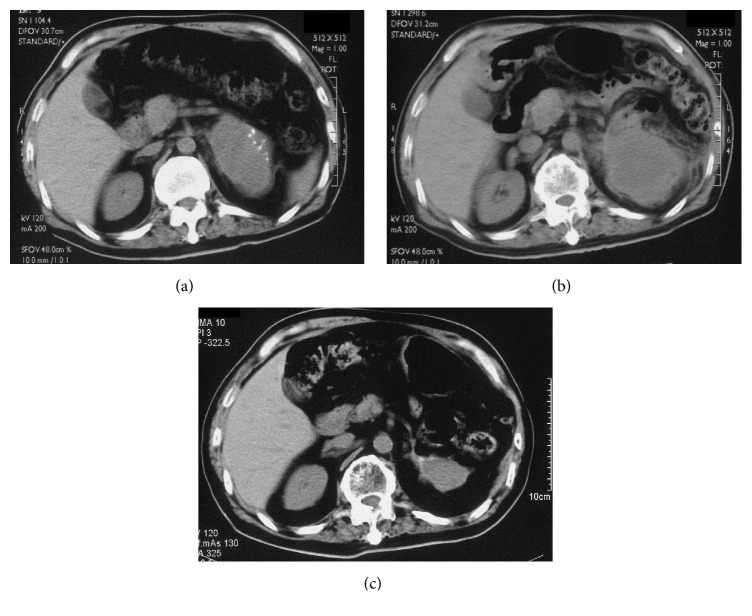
Abdominal computed tomography (CT) image of a left adrenal mass. (a) A preoperative CT scan revealing a 7 × 6 cm tumor with calcification. (b) A CT scan taken 4 months after surgical tumor removal showing a 12 × 9 cm recurrent tumor. (c) Three years after radiotherapy, the residual tumor was 4 × 3 cm.
